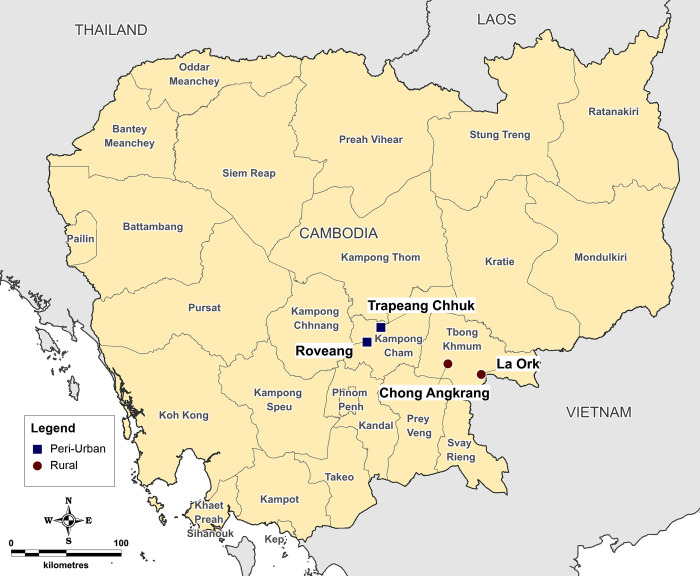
Study site location map. Custom map produced by the authors using MapInfo Professional v15.0.2 (Pitney Bowes Software Inc. 2015, Stamford, CT; https://www.pitneybowes.com) geographic information system mapping software. Site location data were acquired by the study team using global positioning system (GPS). Baseline spatial data were obtained from the GADM database of Global Administrative Areas (https://gadm.org) under a CC-BY 4.0 license [15].
